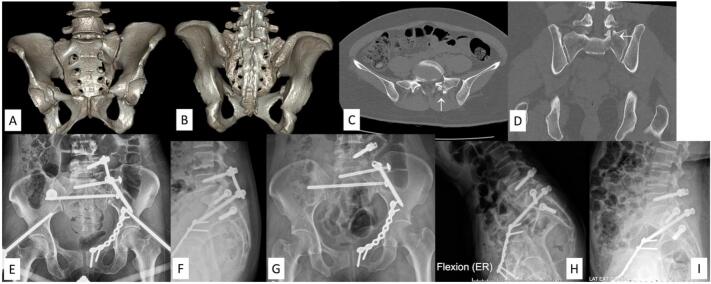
Demonstrating an AO type C1 sacral fracture, Isler subtype 2a (case No. 7). (A-D) Preoperative CT scan and CT reconstruction images showing a longitudinal fracture with a simple intraarticular fracture of the left S1 superior facet (arrow). (E, F) Postoperative radiographs after transiliac-transsacral screw fixation with left triangular fixation. (G-I) Postoperative dynamic radiographs after rod removal with preserved lower lumbar motion.
